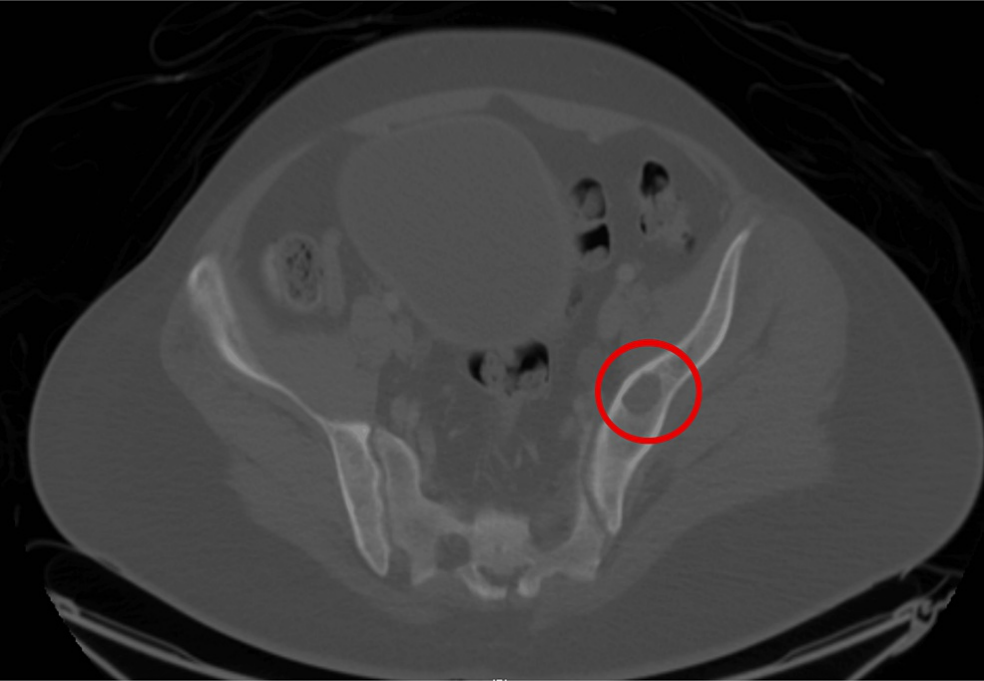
An axial CT scan of the pelvis revealed multiple osteolytic lesions and an acetabular protrusion
